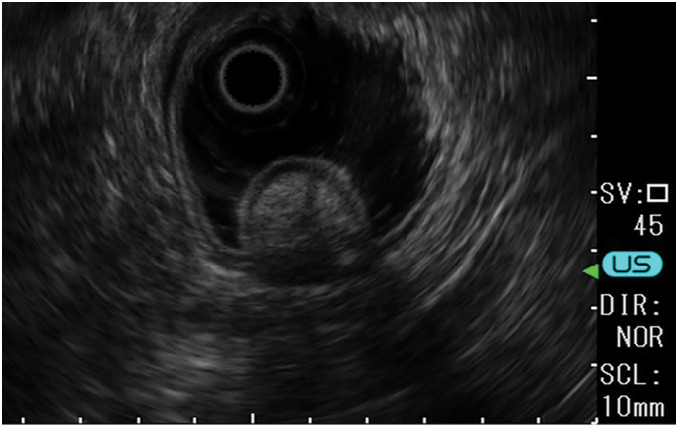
Endoscopic ultrasonography image. The lesion appears as a well-defined hypoechoic mass with the 3rd and 4th layers as the main parts. The lesion is a well-demarcated, hypoechoic mass with 3rd and 4th layers, internal dots of hyperechoic and duct-like echoes, and thickening of the 4th layer.
